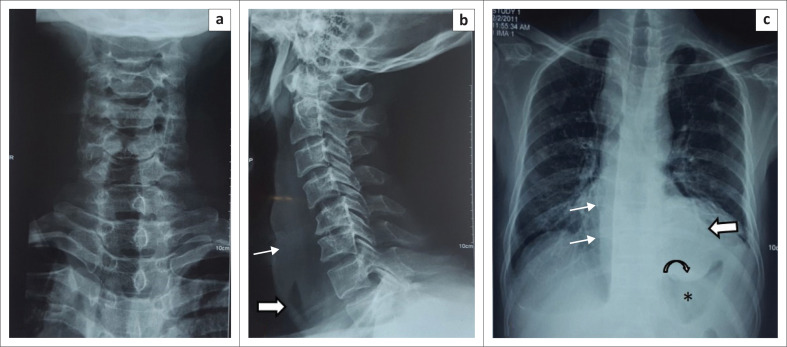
Radiograph of the neck, anteroposterior (a) and lateral (b), demonstrates anterior displacement of the airway and oesophagus (thick arrow) and widening of the prevertebral soft tissue (thin arrow). The frontal chest radiograph (c) indicates displacement of the right paraspinal stripe (thin arrows) and a retrocardiac opacity (thick arrow) indenting (curved arrow) the gastric fundus (asterisk).
